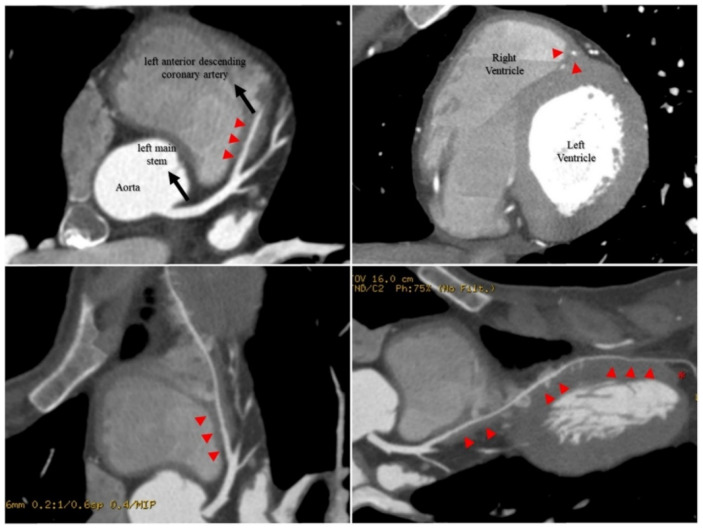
LAD intramyocardial segment shown in CCTA imaging. CCTA revealed an extensive LAD intramyocardial segment (red arrowheads) starting at its proximal segment and running adjacent to the right ventricular free wall in the mid-distal portion, normalizing its usual anatomical position at the apex level (red asterisk). CCTA: coronary computed tomography angiography; LAD: left anterior descending coronary artery.
